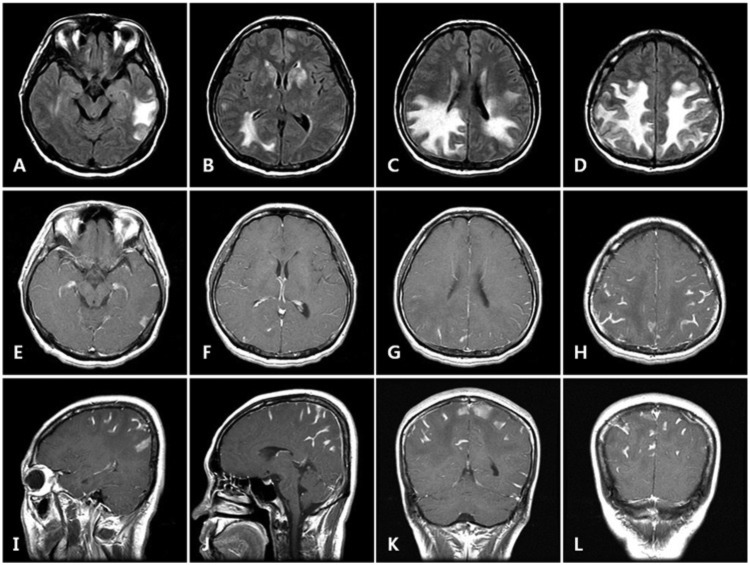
Axial FLAIR (A-D) imaging shows confluent symmetrical hyperintensities in parietal, left temporal, and right occipital white matters. Axial, saggital, and coronal T1WI postcontrast (E-L) imaging shows extensive leptomeningeal enhancement of parietal, temporal, and occipital regions.
